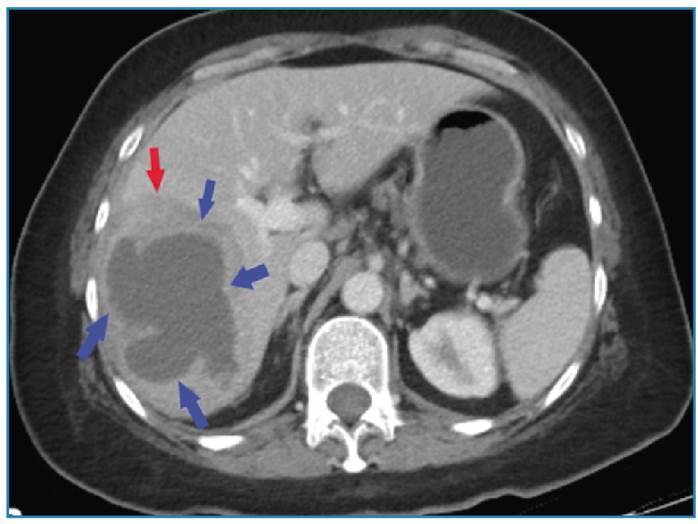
Computed tomography showing a thick-walled hypodense cystic lesion (blue arrows) with surrounding edema (red arrow).
